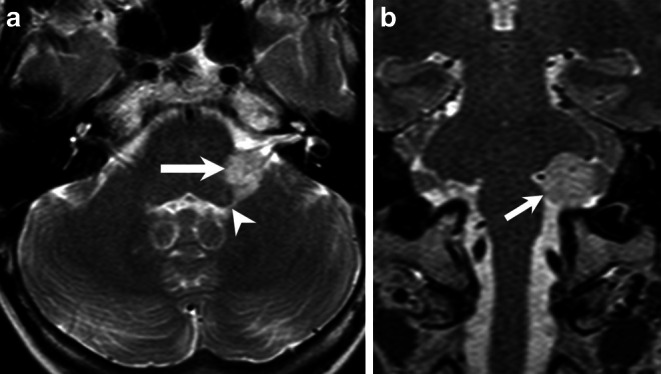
Axial T2-weighted image of the brain (a) shows a space-occupying lesion (*arrow*) located within the left cerebellopontine angle (CPA), encasing the cochlear nerve as well as the inferior vestibular nerve. Mind the small portion of the tumor extending into the foramen of Luschka (*arrowhead*). Coronal T2-weighted image (b) showed the extension of the tumor within the left CPA (*arrow*)
